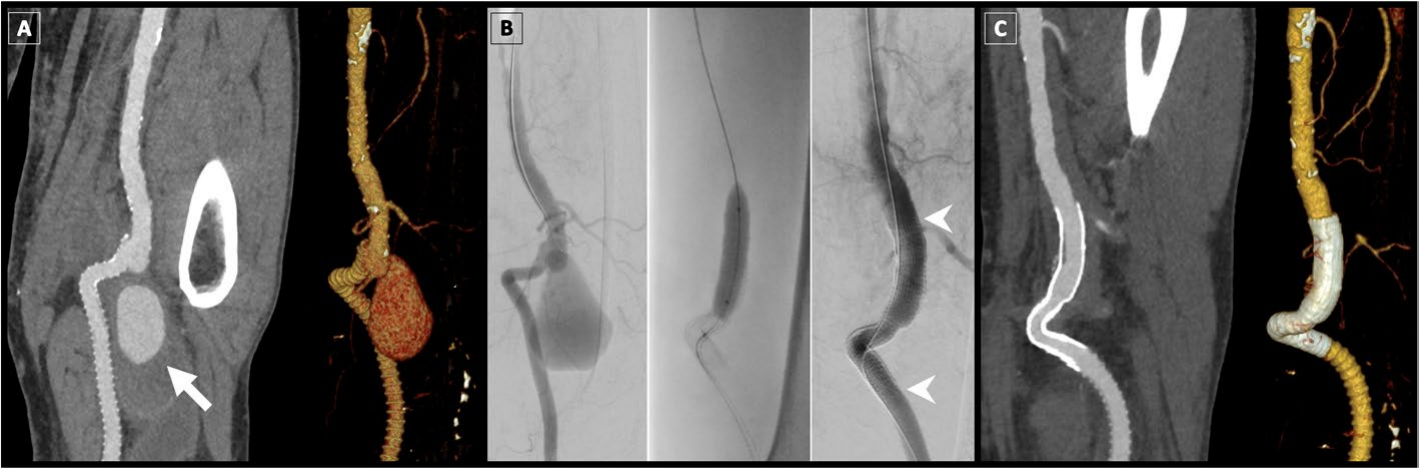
Viabahn endoprosthesis for femoral aneurysm repair. A 77-year-old man with previous P1/P3 bypass operation presented with swelling and pain in the distal posterior aspect of his thigh. A CT angiography (CTA) revealed the presence of a false aneurysm of the distal superficial femoral artery (arrow). B The patient underwent stent graft repair using a Viabahn endoprosthesis (indicated by arrowheads). The successful exclusion of the aneurysm with preservation of blood flow was confirmed by final target site angiography. C Follow-up CTA at 1 year demonstrated sustained preservation of blood flow
